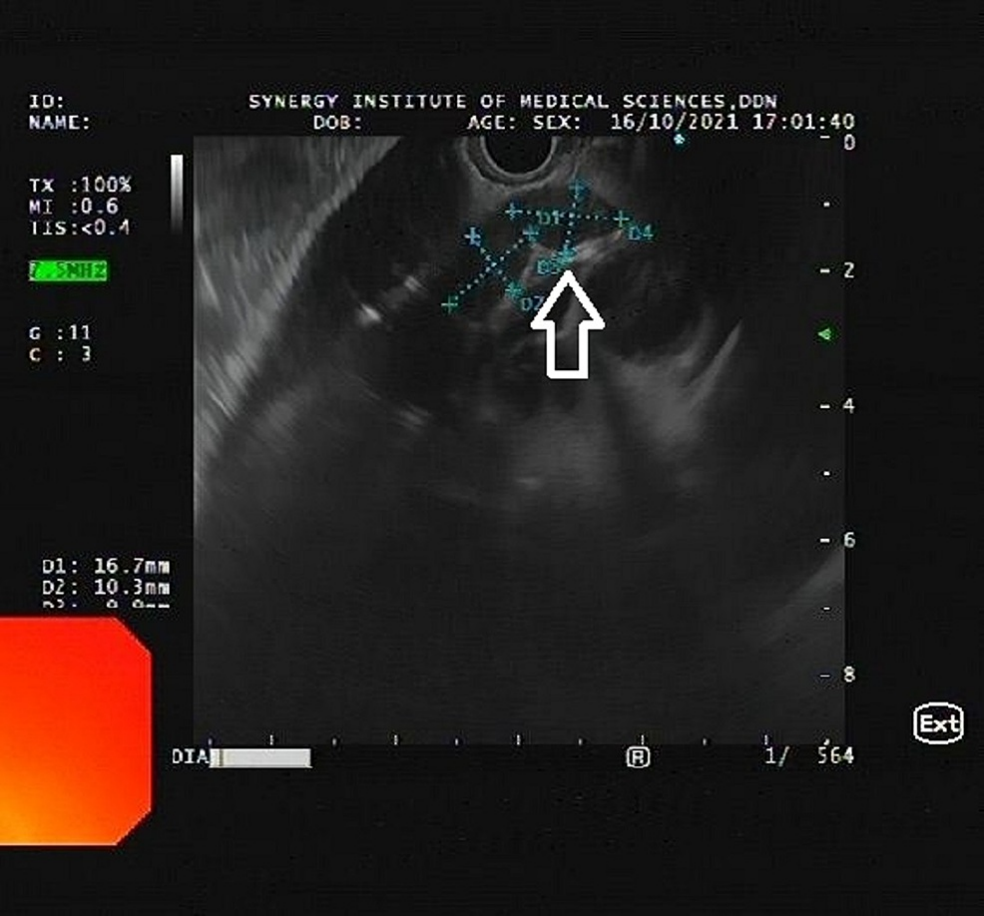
Endoscopic ultrasound showing the enlarged mediastinal lymph nodes (arrow).
